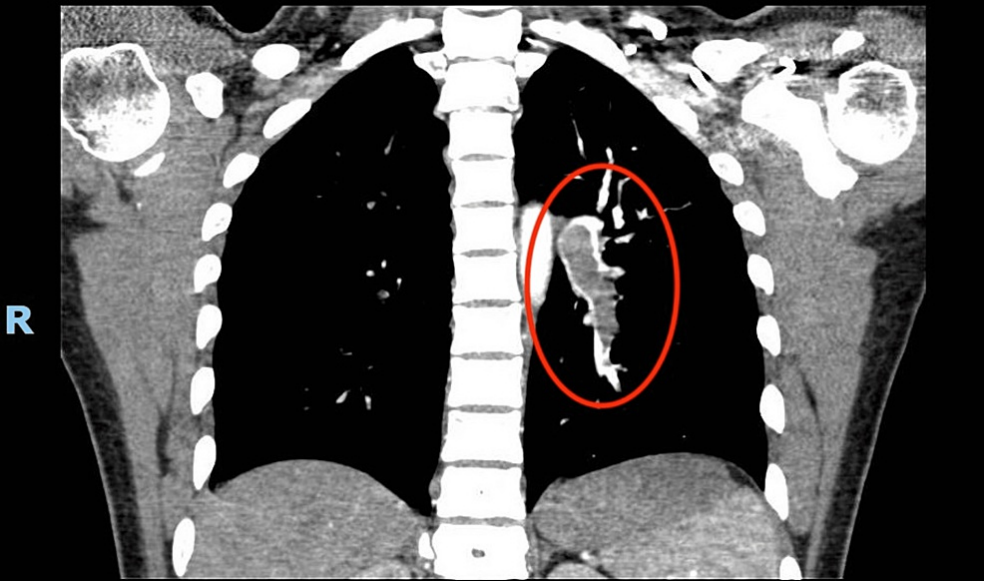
A CT angiogram of the chest (coronal) showing extensive involvement (noted within the red circle) of the left pulmonary artery and subsequent segmental and interlobar arteries.
